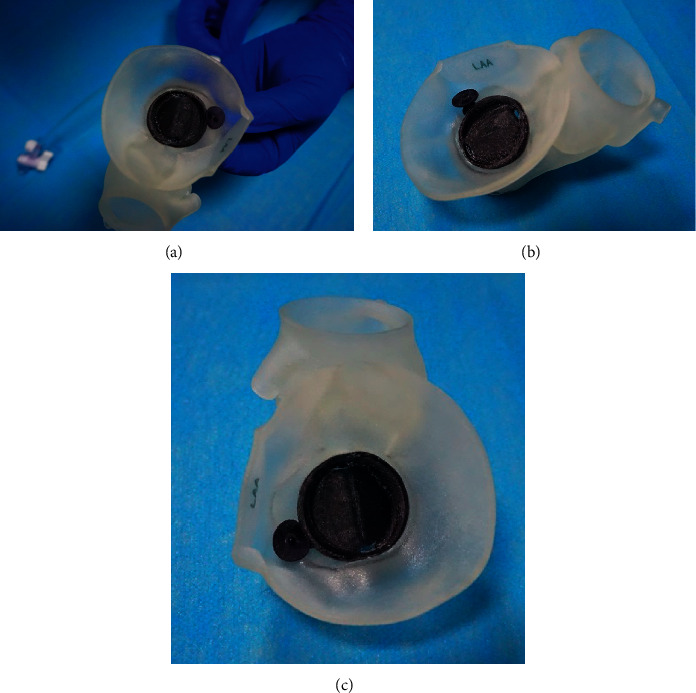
The 3D printing model of patients was made based on the postoperative CT results. The operator observed the position of the occluder device in vitro and evaluated the results of the operation.
